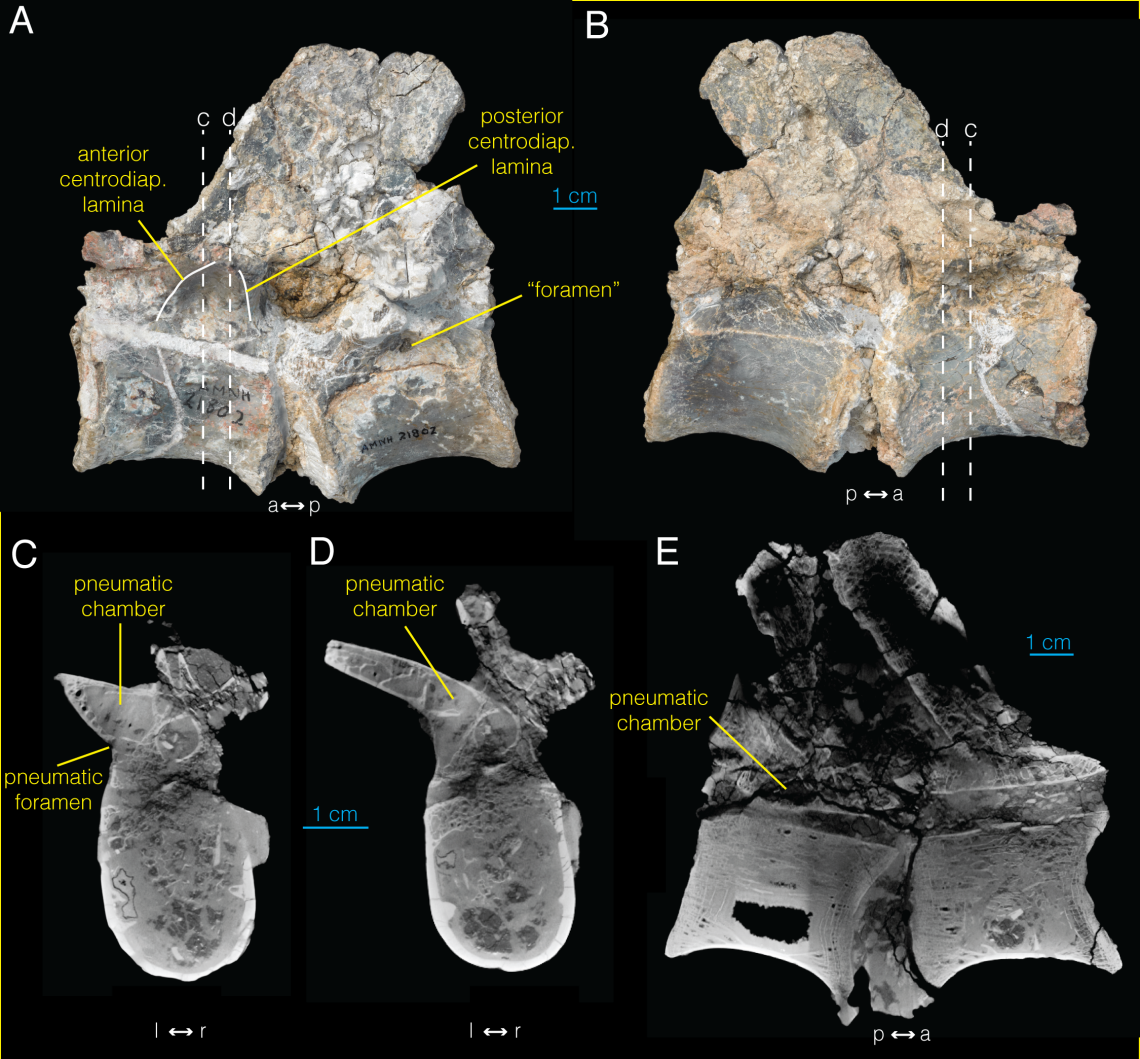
Proximal caudal vertebrae of *Archaeornithomimus* (AMNH FARB 21802) and associated CT images. A, left lateral view; B, right lateral view; C, D, select transverse sections; E, midsagittal section. Dashed lines and associated letters indicate location and letter designation of CT image slices.
